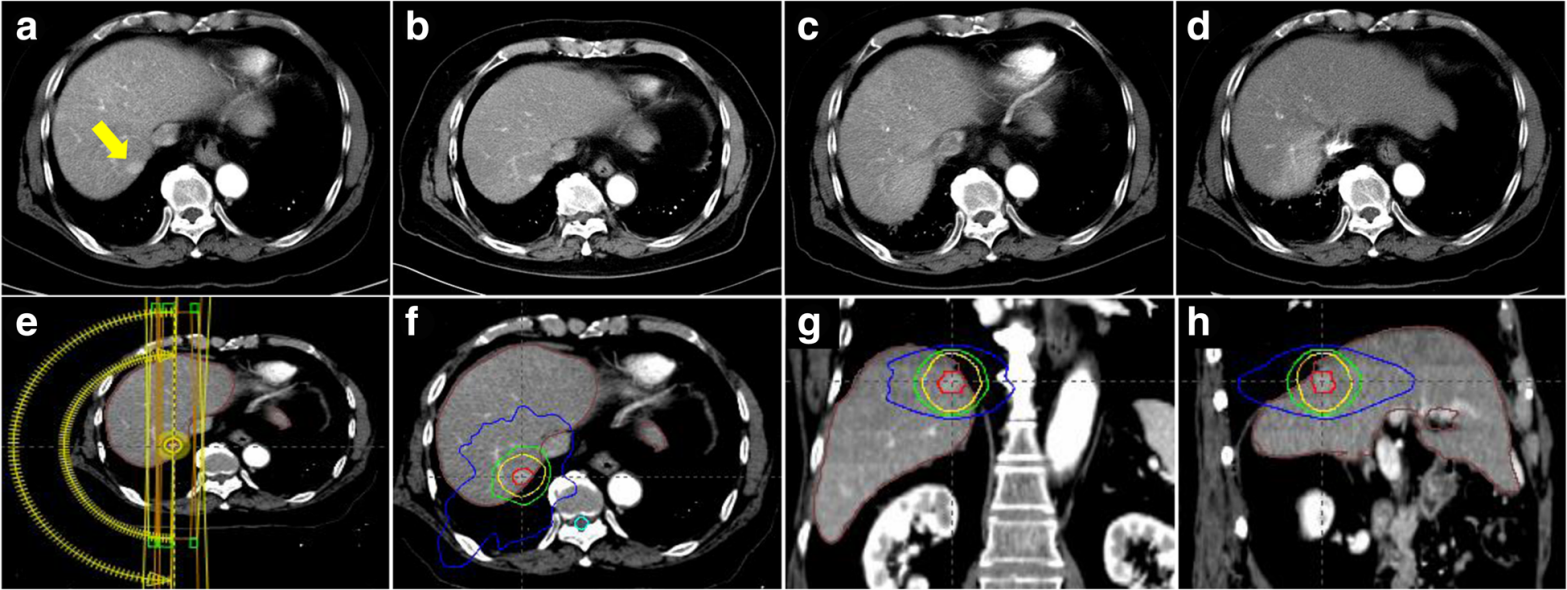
SBRT using a respiratory-gated VMAT technique. A representative patient with HCC (located in segment 7) who achieved complete response at 6 months after SBRT. CT scans in arterial phase before SBRT (a), 1 month (b), 3 months (c), and 6 months (d) after SBRT, respectively. CT scans for VMAT plan which shows 2 arc beams (e) and isodose lines in axial (f), coronal (g), and sagittal (h) views. The red line is the gross tumor volume, and yellow, green, and blue lines are the 97, 70, and 30% isodose lines, respectively
